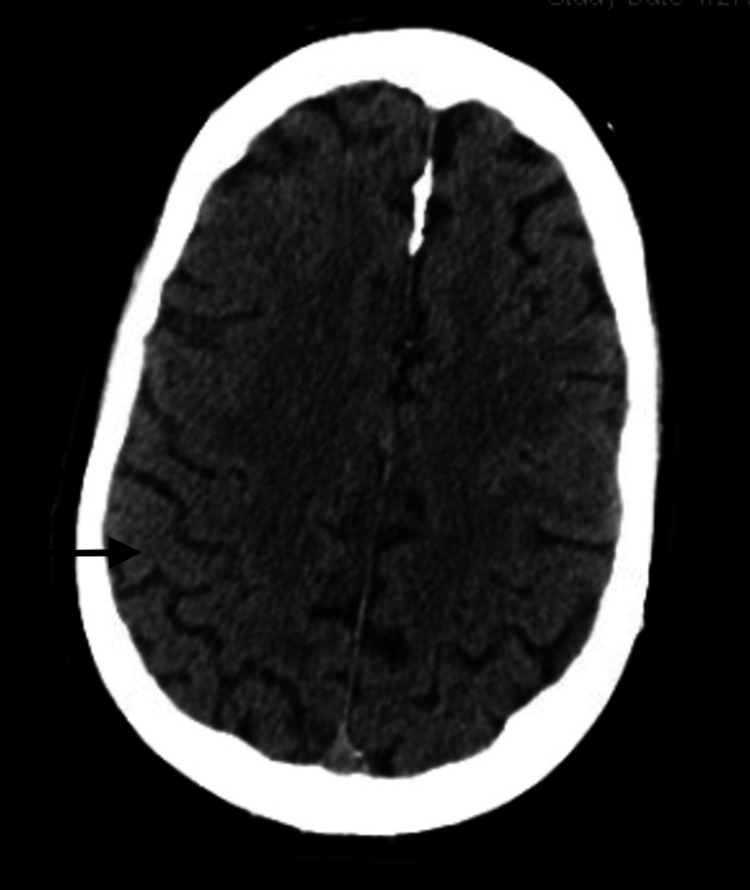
Computed tomography scan of the brain showing complete resolution of subdural hematoma (see black arrow)
